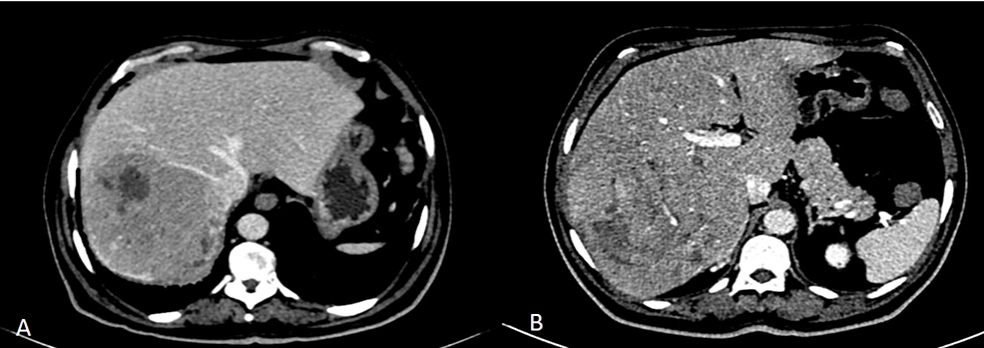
Preoperative CECT A: right hepatic vein abutting the tumor; B: right posterior branch of the right portal vein going into the tumor CECT: contrast-enhanced computed tomography
